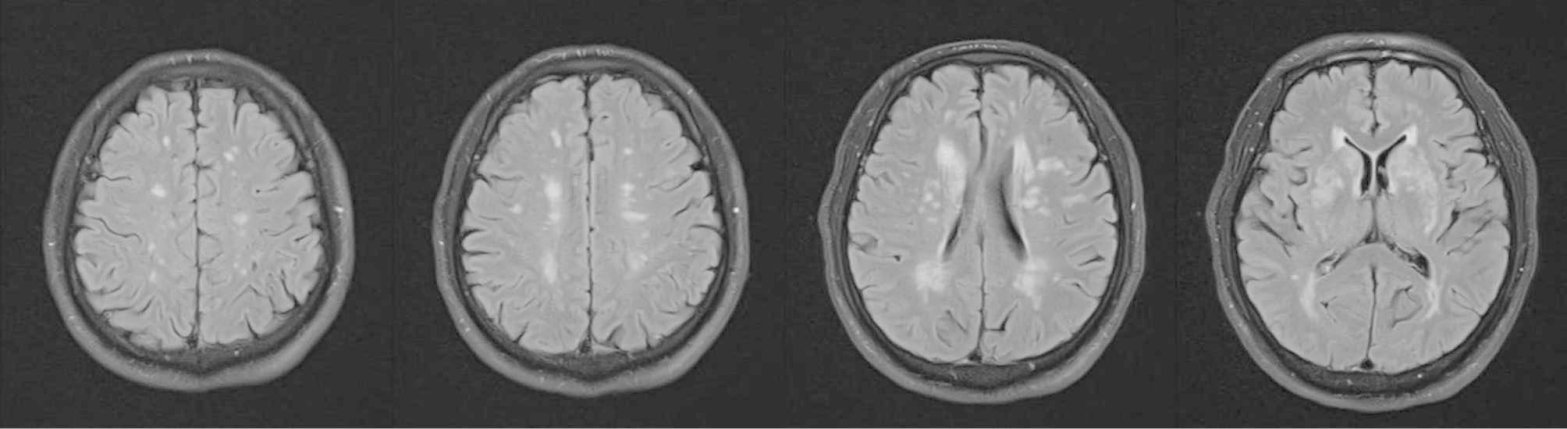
A 54-year-old IVS4 FD patient without hypertension suffering from advanced FD leukoencephalopathy. Serial axial FLAIR images demonstrated heavy white matter burden at periventricular and deep white matter regions. Large areas of patchy and confluent T2/FLAIR hyperintense signals were appreciated
